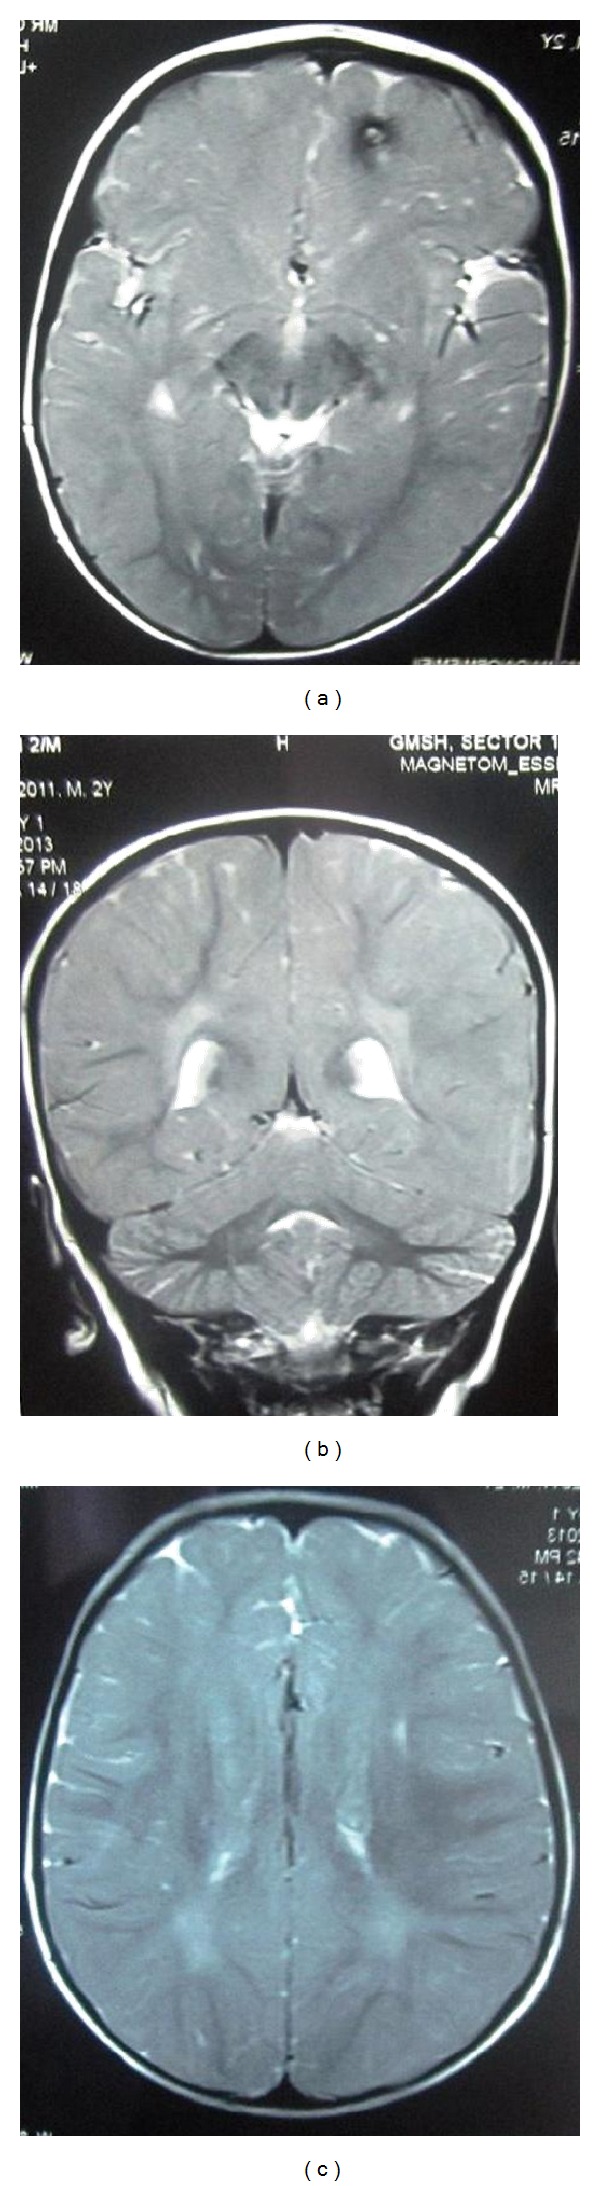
Magnetic resonance imaging of the brain. (a) T2 weighted axial section showing small hypointense focal lesion in left frontal lobe suggestive of cerebral contusion. (b) T2 weighted coronal section and (c) T2 weighted axial section showing ill-defined patchy areas of hyperintense signal in bilateral peritrigonal and paraventricular parietal white matter.
